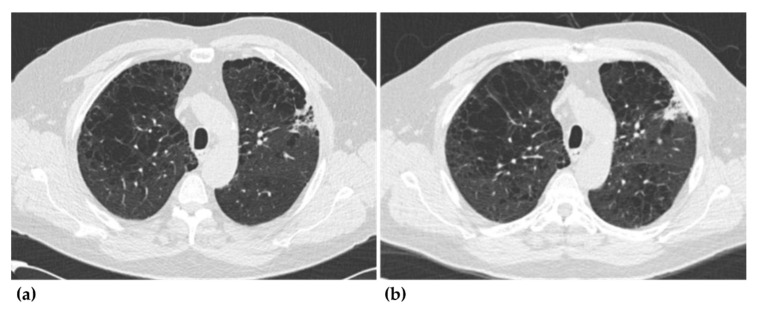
The only false-negative case reported by the deep-learning based computer-aided diagnosis system. On the CT examination of the first screening round, the lesion in the left upper lobe (as shown in image (a) was not detected by the radiologist involved in the national lung screening trial. The CT examination of the second screening round showed an enlarged consolidative mass (as shown in image (b), and the lesion was confirmed as lung cancer (adenocarcinoma).
